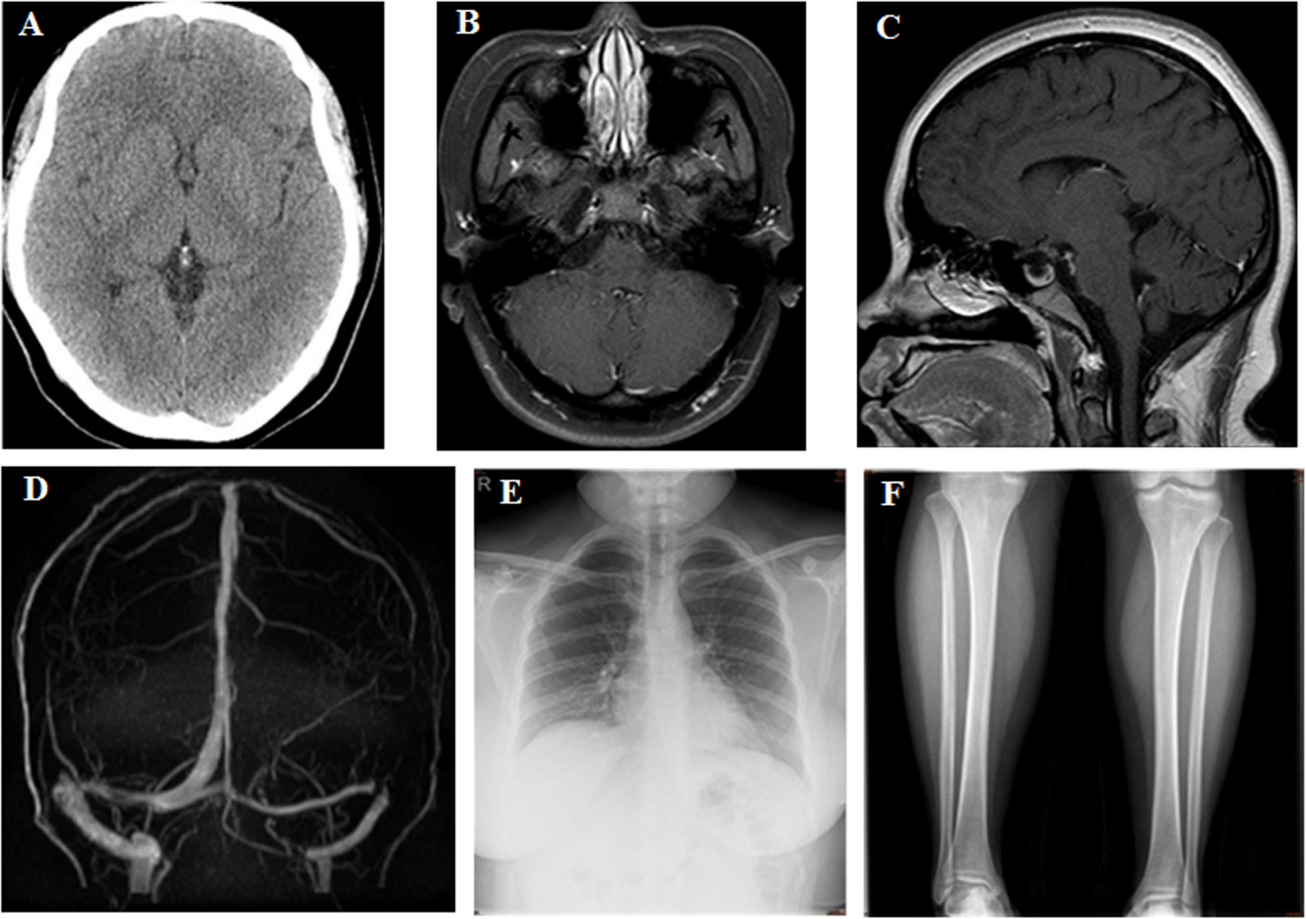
A Computed tomography (CT) scan image of the head. B T1-weighted image with contrast (T1W + C) shows a visible water signal in front of the cerebellar hemisphere without gadolinium enhancement. C T1W + C image exhibits subtle leptomeningeal enhancement. D MRV image reveals the right dominant type of venous drainage system and right superior sigmoid sinus segment diverticulum. E X-ray image of the chest. F X-ray image of legs plain
